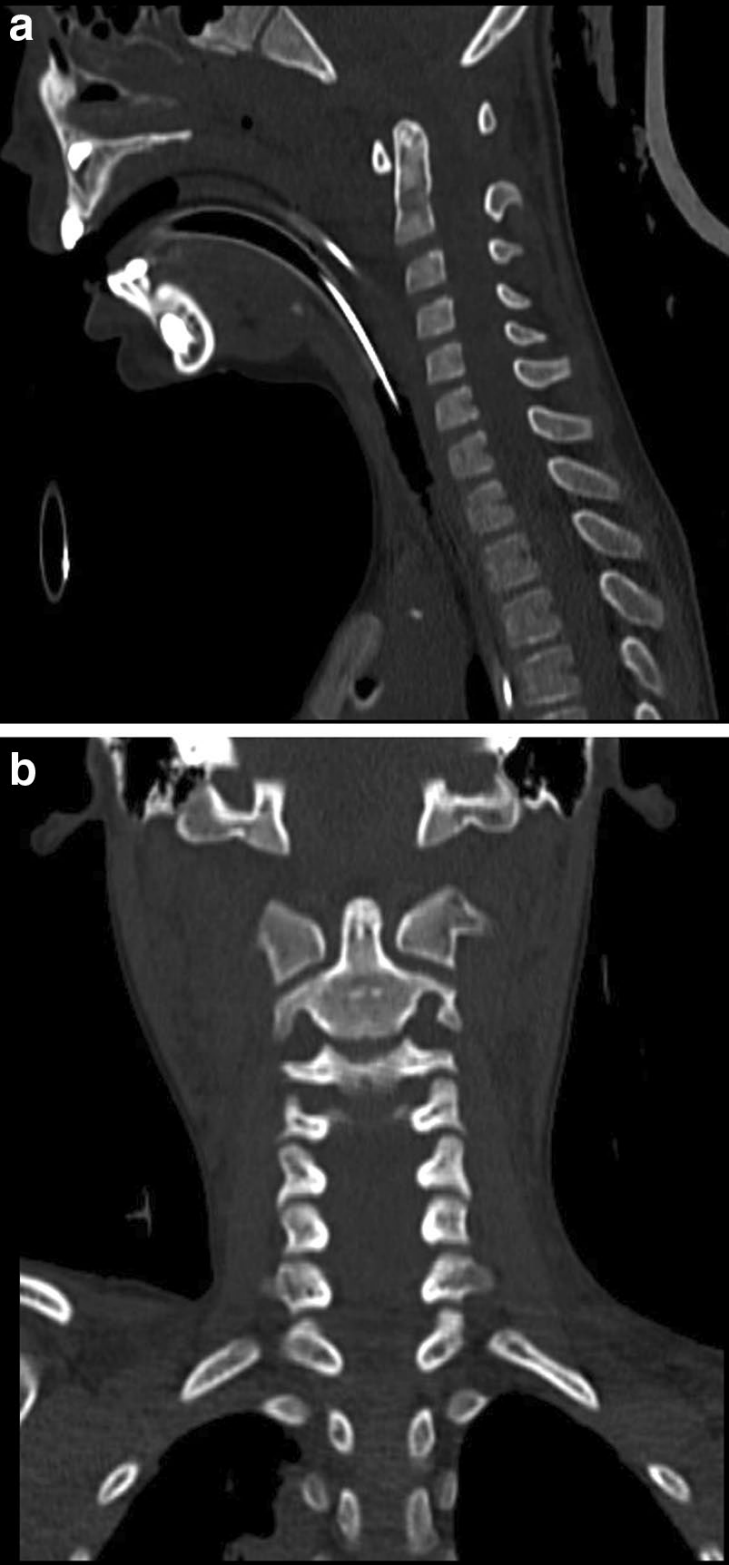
CT showing atlanto-occipital dislocation: a coronal; and b sagittal. Case courtesy of Dr Yair Glick, Radiopaedia.org, rID: 52910
